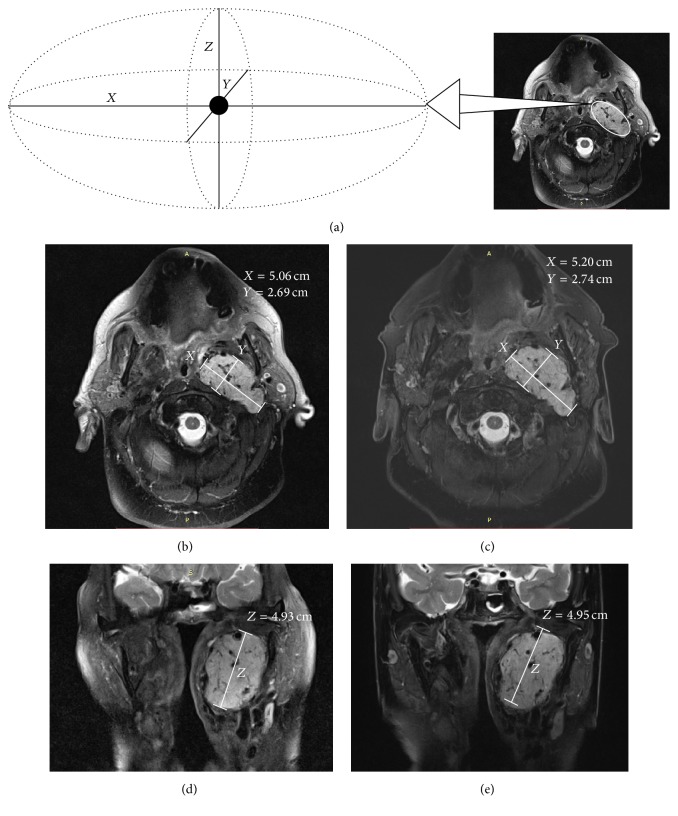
(a) The untreated left glomus vagale was considered to have an ellipsoid shape. The 3 perpendicular dimensions in the anteroposterior (*X*), mediolateral (*Y*), and craniocaudal (*Z*) directions were used to calculate the maximum axial area and volume. ((b)–(e)) Serial MRI scans (*T*
